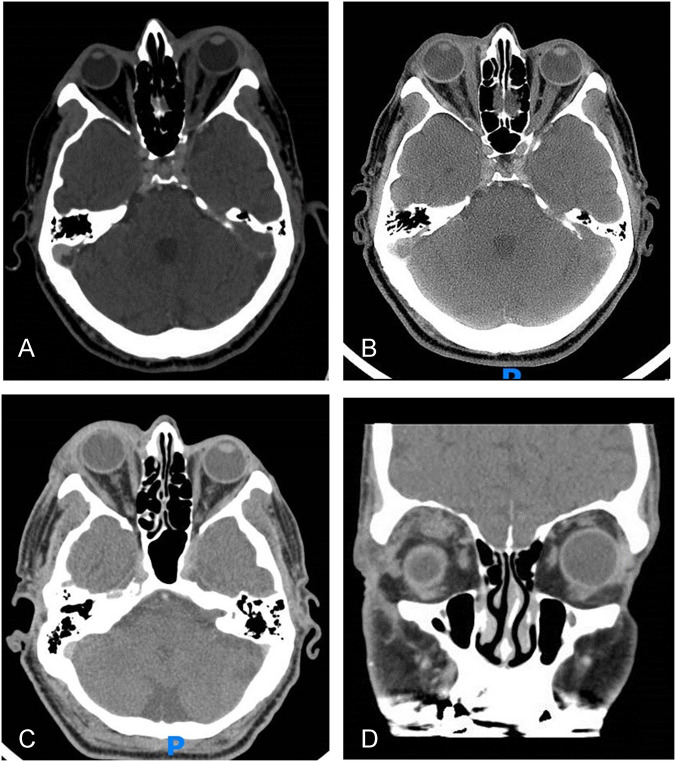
case number 2, CT scans in different presentations. A First presentation (March 2017) shows proptosis of the left eye, preseptal swelling, medial rectus and lacrimal gland enlargement. B Presentation (February 2018), right eye proptosis and medial rectus enlargement. C, D presentation (December 2018), axial scan (C) demonstrates right proptosis, preseptal swelling and lacrimal gland enlargement. Coronal scan (D) shows superior rectus swelling accompanied with infiltration the surrounding fat.
